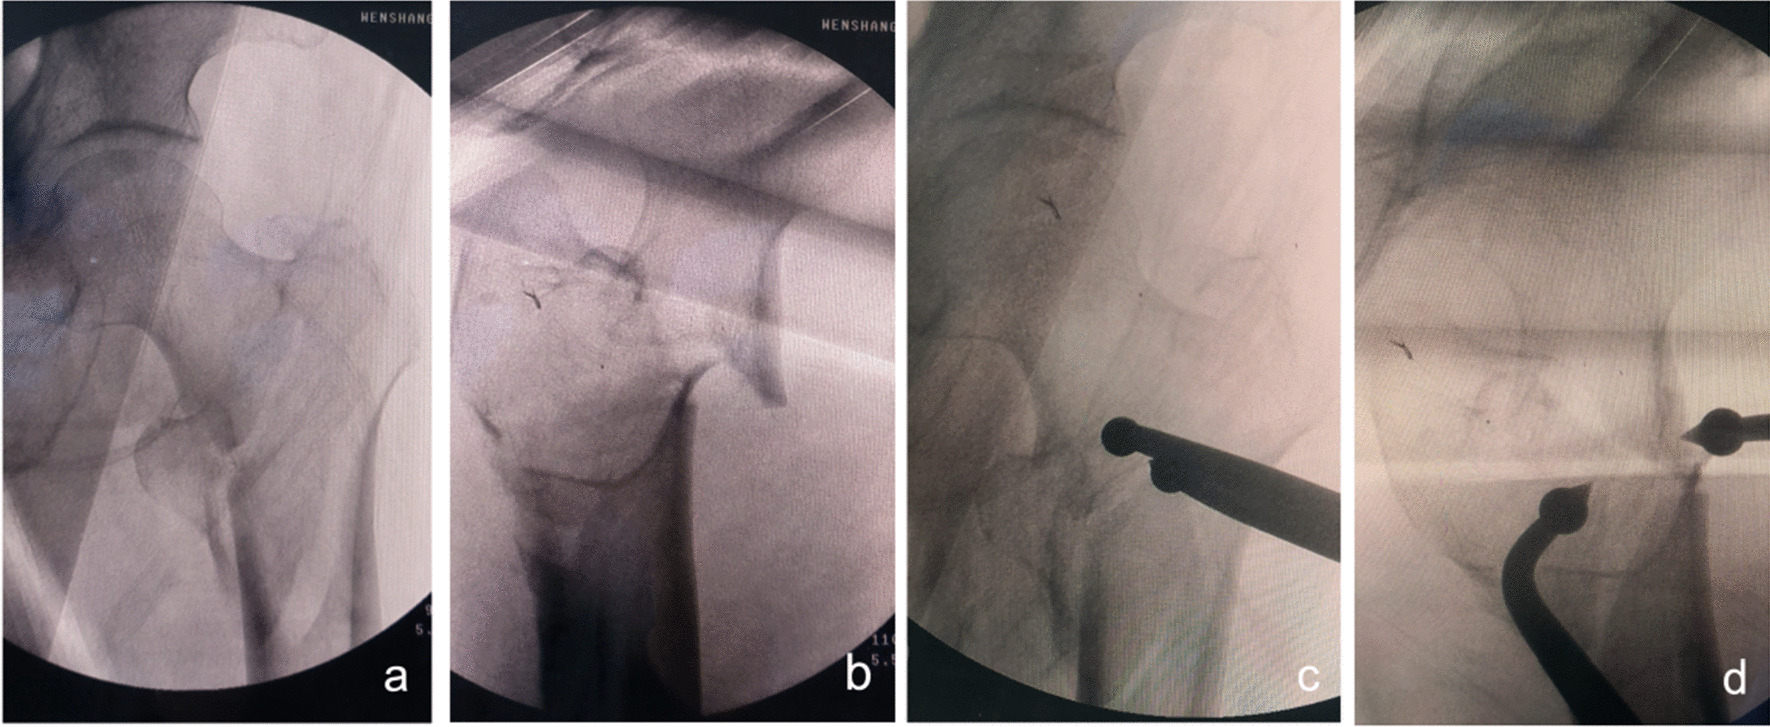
Anteroposterior and lateral X-ray films before and after reduction in patients with difficulty in reduction on the sagittal plane and pronation displacement of the proximal fracture segment a, b Before reduction; c, d After reduction (one head placed in front of the proximal fracture segment and the other head behind the greater trochanter)
